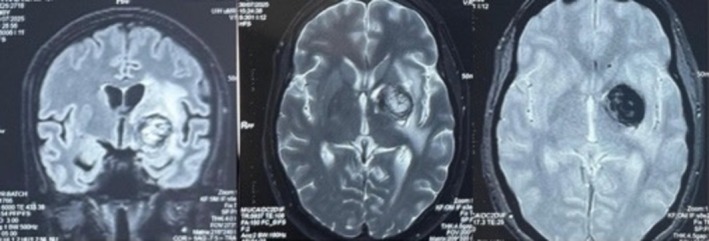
MRI (SWI sequence) at 3‐month follow‐up showing blooming artifact due to coils in the basal ganglia region with no new infarcts or hemorrhage.
